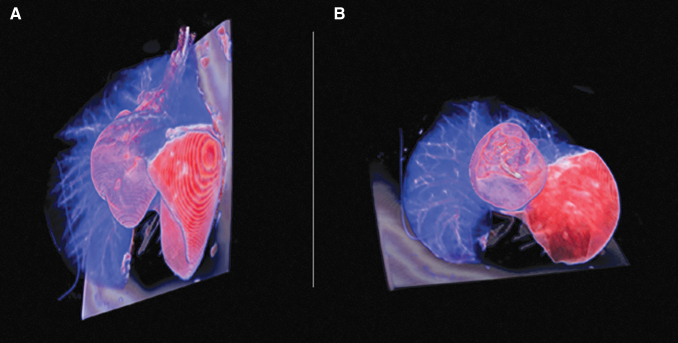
Three-dimensional rendering of 18F-FDG-labeled placental stem cells delivered in the left lower lobe. (A) Perspective view. (B) Bottom view. Fused PET-CT image reconstruction showing in blue the normal lung parenchyma, and in *red* the isotope-labeled cells distributed across the entire left lower lobe.
